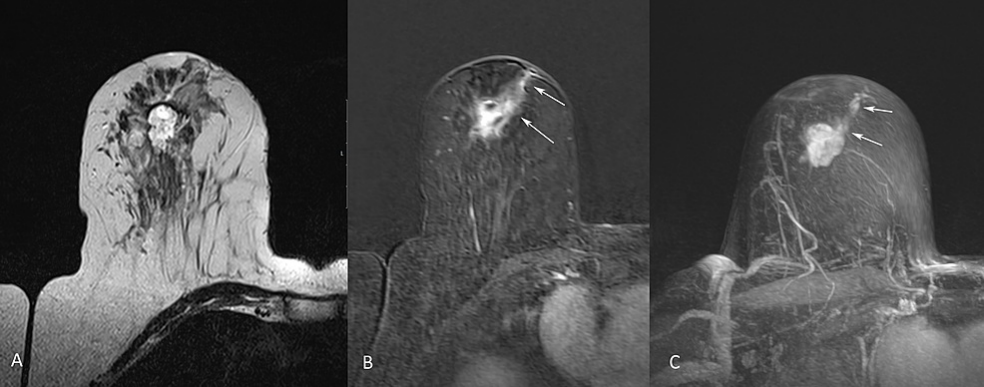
MR images of the right breast (A) Pre-contrast T2-weighted MR image of the right breast shows a high signal intensity mass in the retro-areolar area in keeping with the biopsy-proven invasive mucinous carcinoma. (B) Axial and (C) maximum intensity projection (MIP) contrast-enhanced subtracted images of the right breast show the enhancing index mass with nodular enhancement along the biopsy needle tract (arrows).
